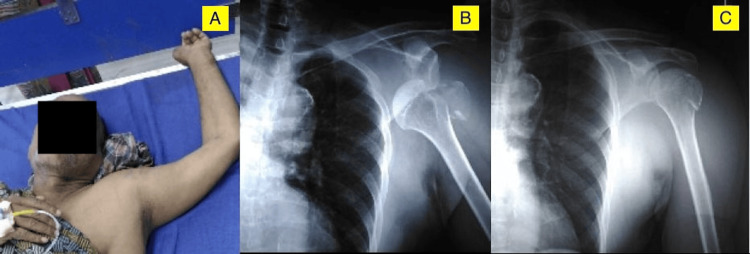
Case 2 A) The left arm and shoulder are in a hyper-abducted position with inferior shoulder fracture-dislocation. B) Radiograph showing left sub-coracoid inferior shoulder fracture-dislocation. C) Post-reduction radiograph showing shoulder in situ after 'two-step manoeuvre'.
